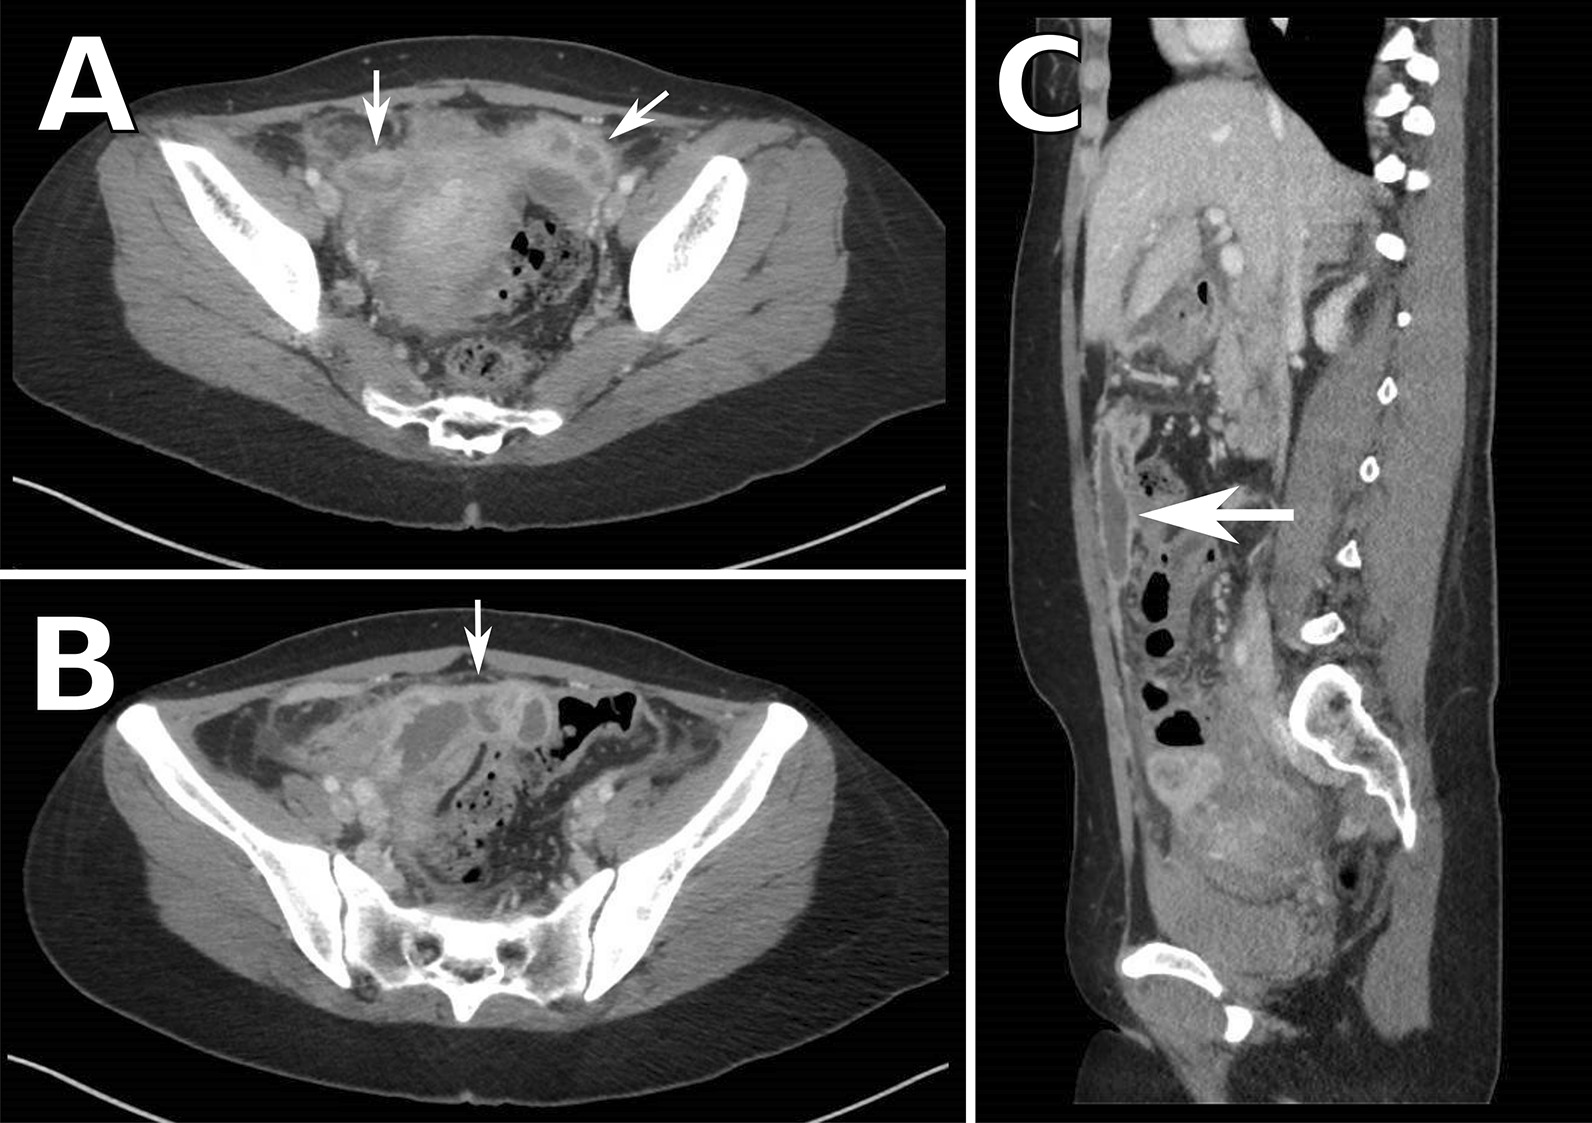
Computed tomography (CT) of abdomen/pelvis demonstrating tubo-ovarian and peritoneal abscesses. A Dilated, fluid-filled fallopian tubes with rim enhancement and thickening with B an associated tubo-ovarian abscess. C Peritoneal abscesses in the anterior mid and left mid-abdomen and pelvis
